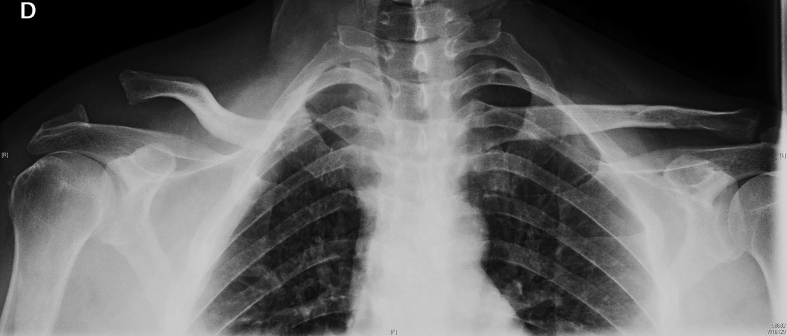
Initial AP X-ray that shows a Rockwood type IV acromioclavicular joint dislocation, and possible implication of sternoclavicular joint.
